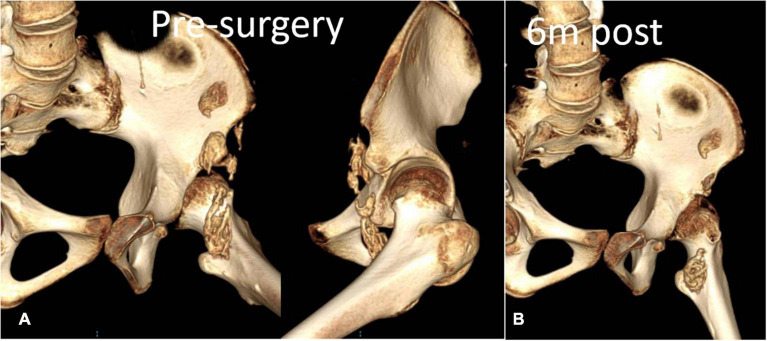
Anteroposterior view of the Computed tomography scan of the left hip confirmed aforementioned findings and additionally revealed heterotopic ossification of the iliopsoas muscle. (A) Preoperative CT reconstruction. (B) 6-month follow-up.
